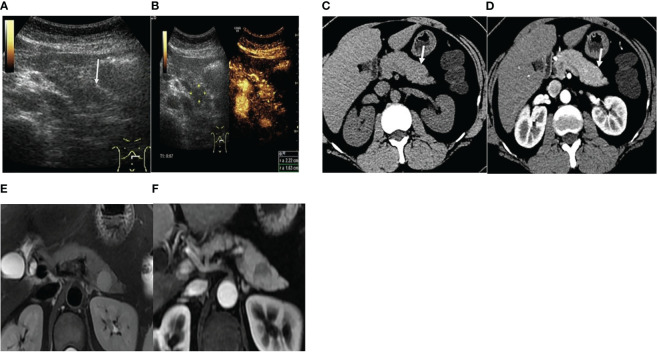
Case 1 in our hospital. (A) Conventional two-dimensional ultrasonography revealed an ill-defined suborbicular lesion in the pancreatic tail (white row), with uneven hypoecho, of approximately 2.2 cm in size. (B) CEUS revealed hyperenhancement in the arterial phase. (C, D) Contrast-enhanced CT demonstrated a round iso-dense lesion (white rows) in the tail of the pancreas, with the mild enhancement in the arterial phase. (E) T2WI sequence revealed a well-defined round mass in the pancreatic tail, with high intensity. (F) Contrast-enhanced MRI showed slightly delayed enhancement (white row).
